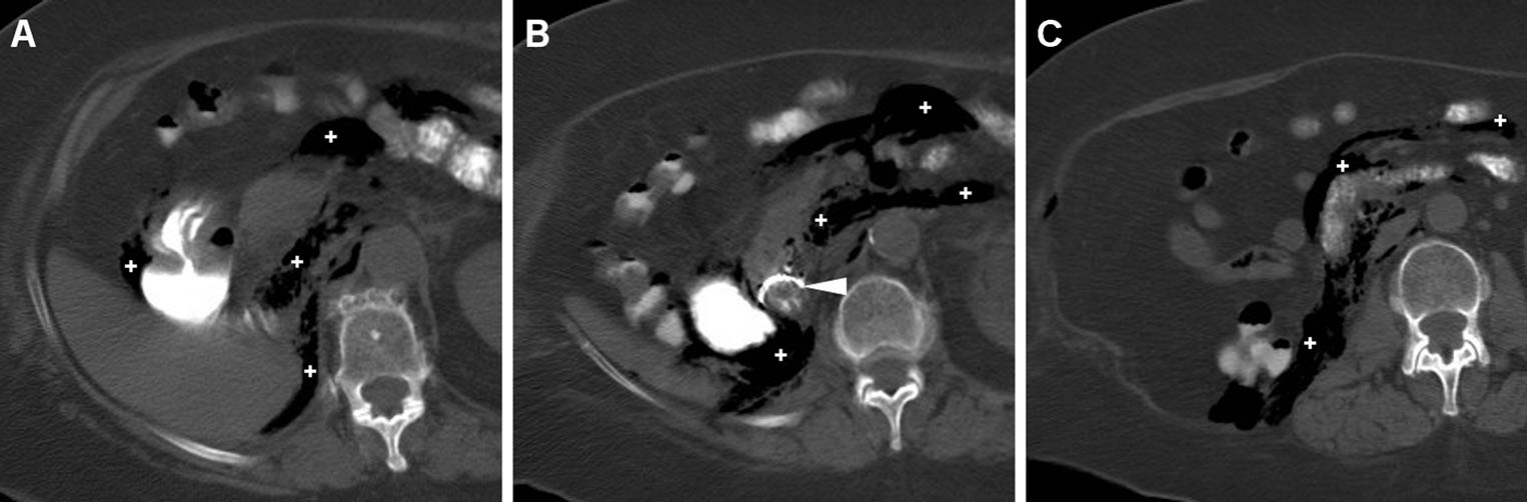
During endoscopic retrograde cholangiopancreatography (*ERCP*), a 65-year-old female with sphincter of Oddi dysfunction experienced duodenal perforation (*DP*), which was recognised intraprocedurally and treated with endoluminal clipping. Twenty-four hours later, unenhanced multidetector CT obtained after peroral ingestion of enteral contrast medium (*CM*) and viewed at the bone window setting showed a well-opacified gastroduodenal lumen without extraluminal CM leak, consistent with sealed DP. Note the persistent extensive retroperitoneal air (+) and metallic clips (*arrowhead in B*)
